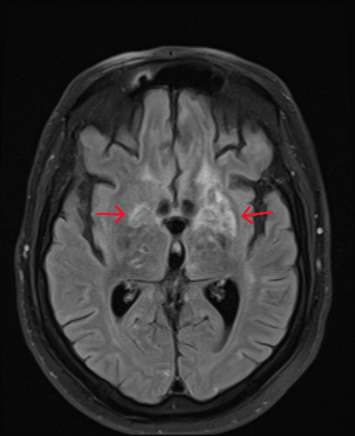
MRI of the brain with and without contrast demonstrates that many of the previously enhancing lesions now show no enhancement. The persisting lesions (red arrows) exhibit reduced enhancement and edema compared to earlier imaging, indicating a partial response to therapy.
